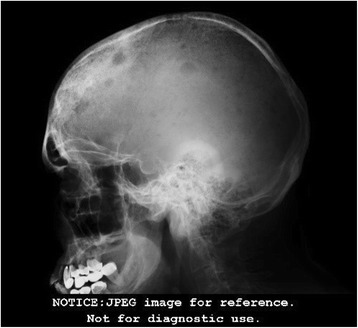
Lateral X-ray of the head. Multiple lytic lesions in the skull
